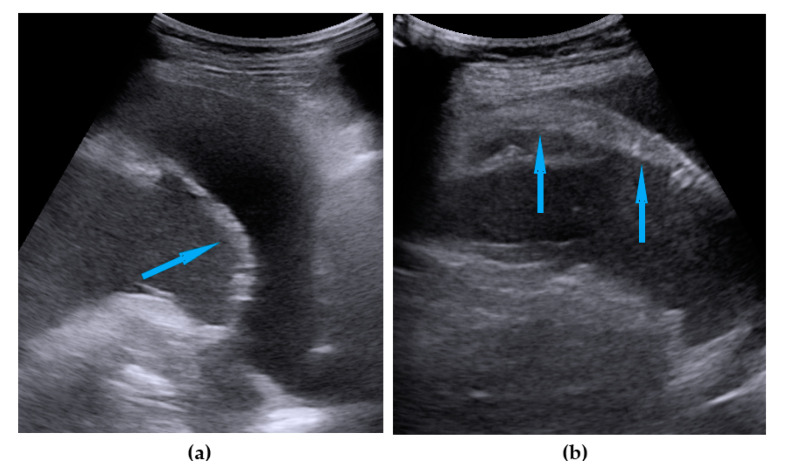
Ultrasound images of left diaphragm peritoneum involvement: (a) nodules; (b) diaphragm thickening. Blue arrows identify lesions.
